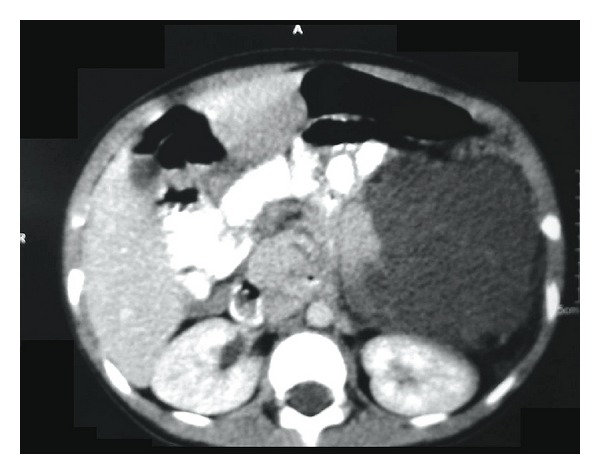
CT of the abdomen showing a 30 cm × 10 cm left sided isodense retroperitoneal mass.
